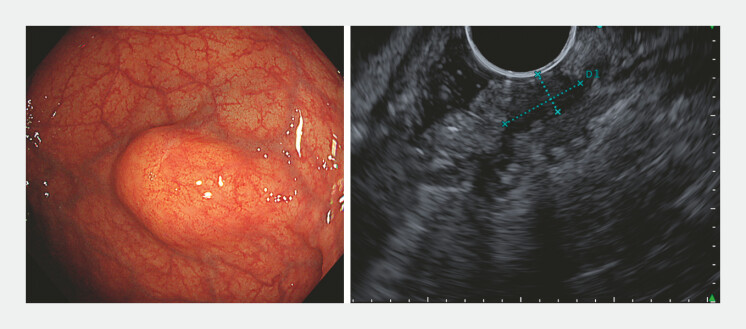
A 15-mm submucosal tumor located in the lower rectum. Endoscopic ultrasonography showing an 11-mm extramural hypoechoic lesion.
